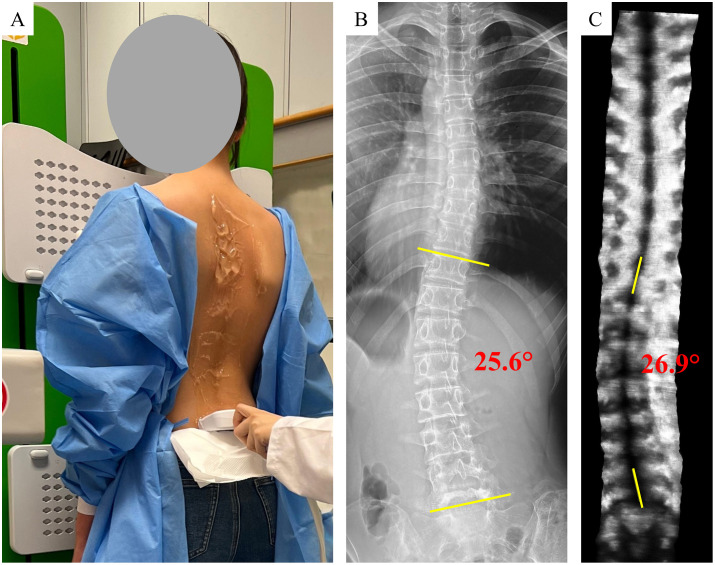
Scoliosis assessment in this study: A) ultrasound scan using the Scolioscan® system; B) standard posteroanterior radiograph showing a Cobb angle of 25.6°; C) corresponding coronal ultrasound image showing a curvature angle of 26.9° using SP method.
